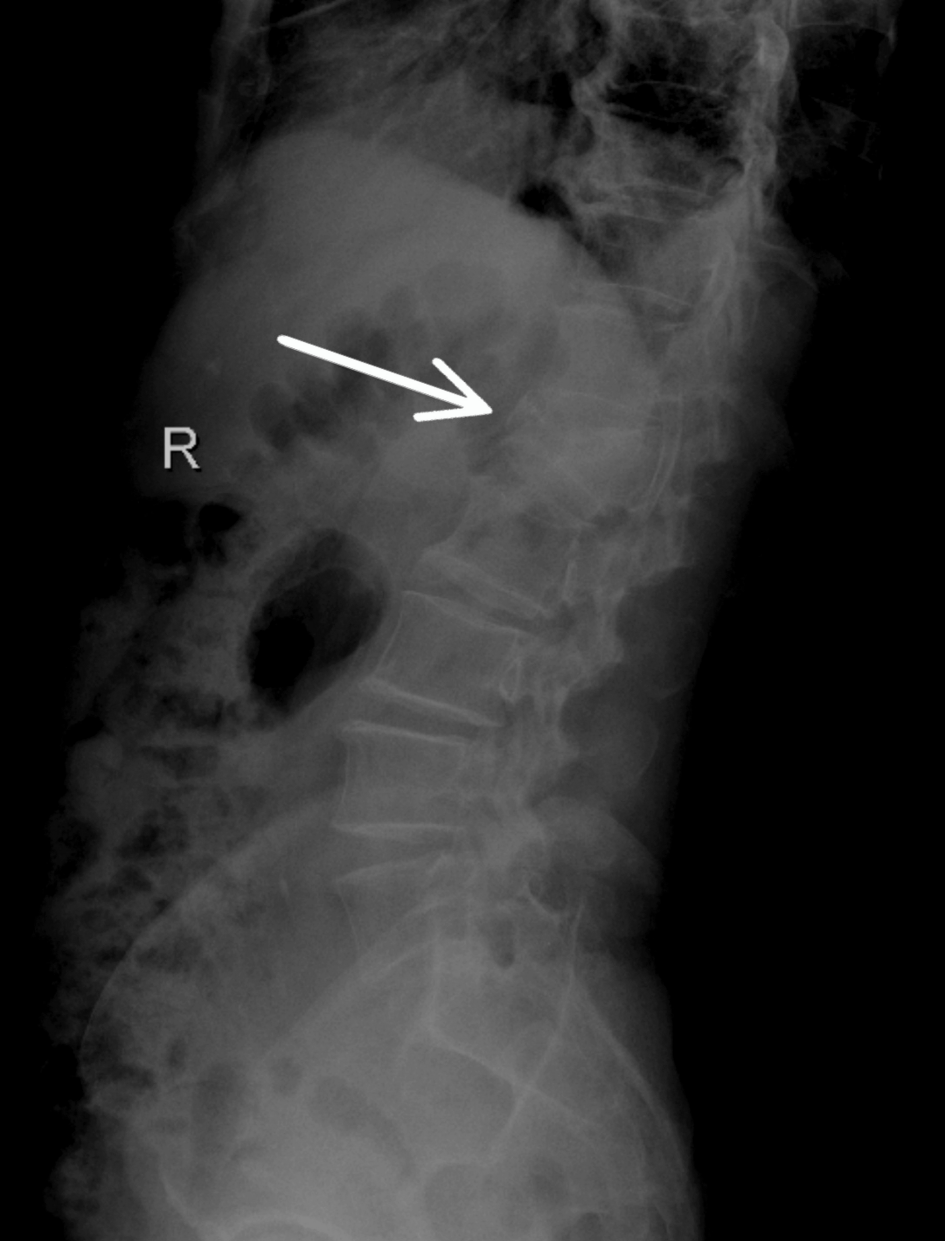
Lateral X-ray view showing L1 vertebral fracture.
